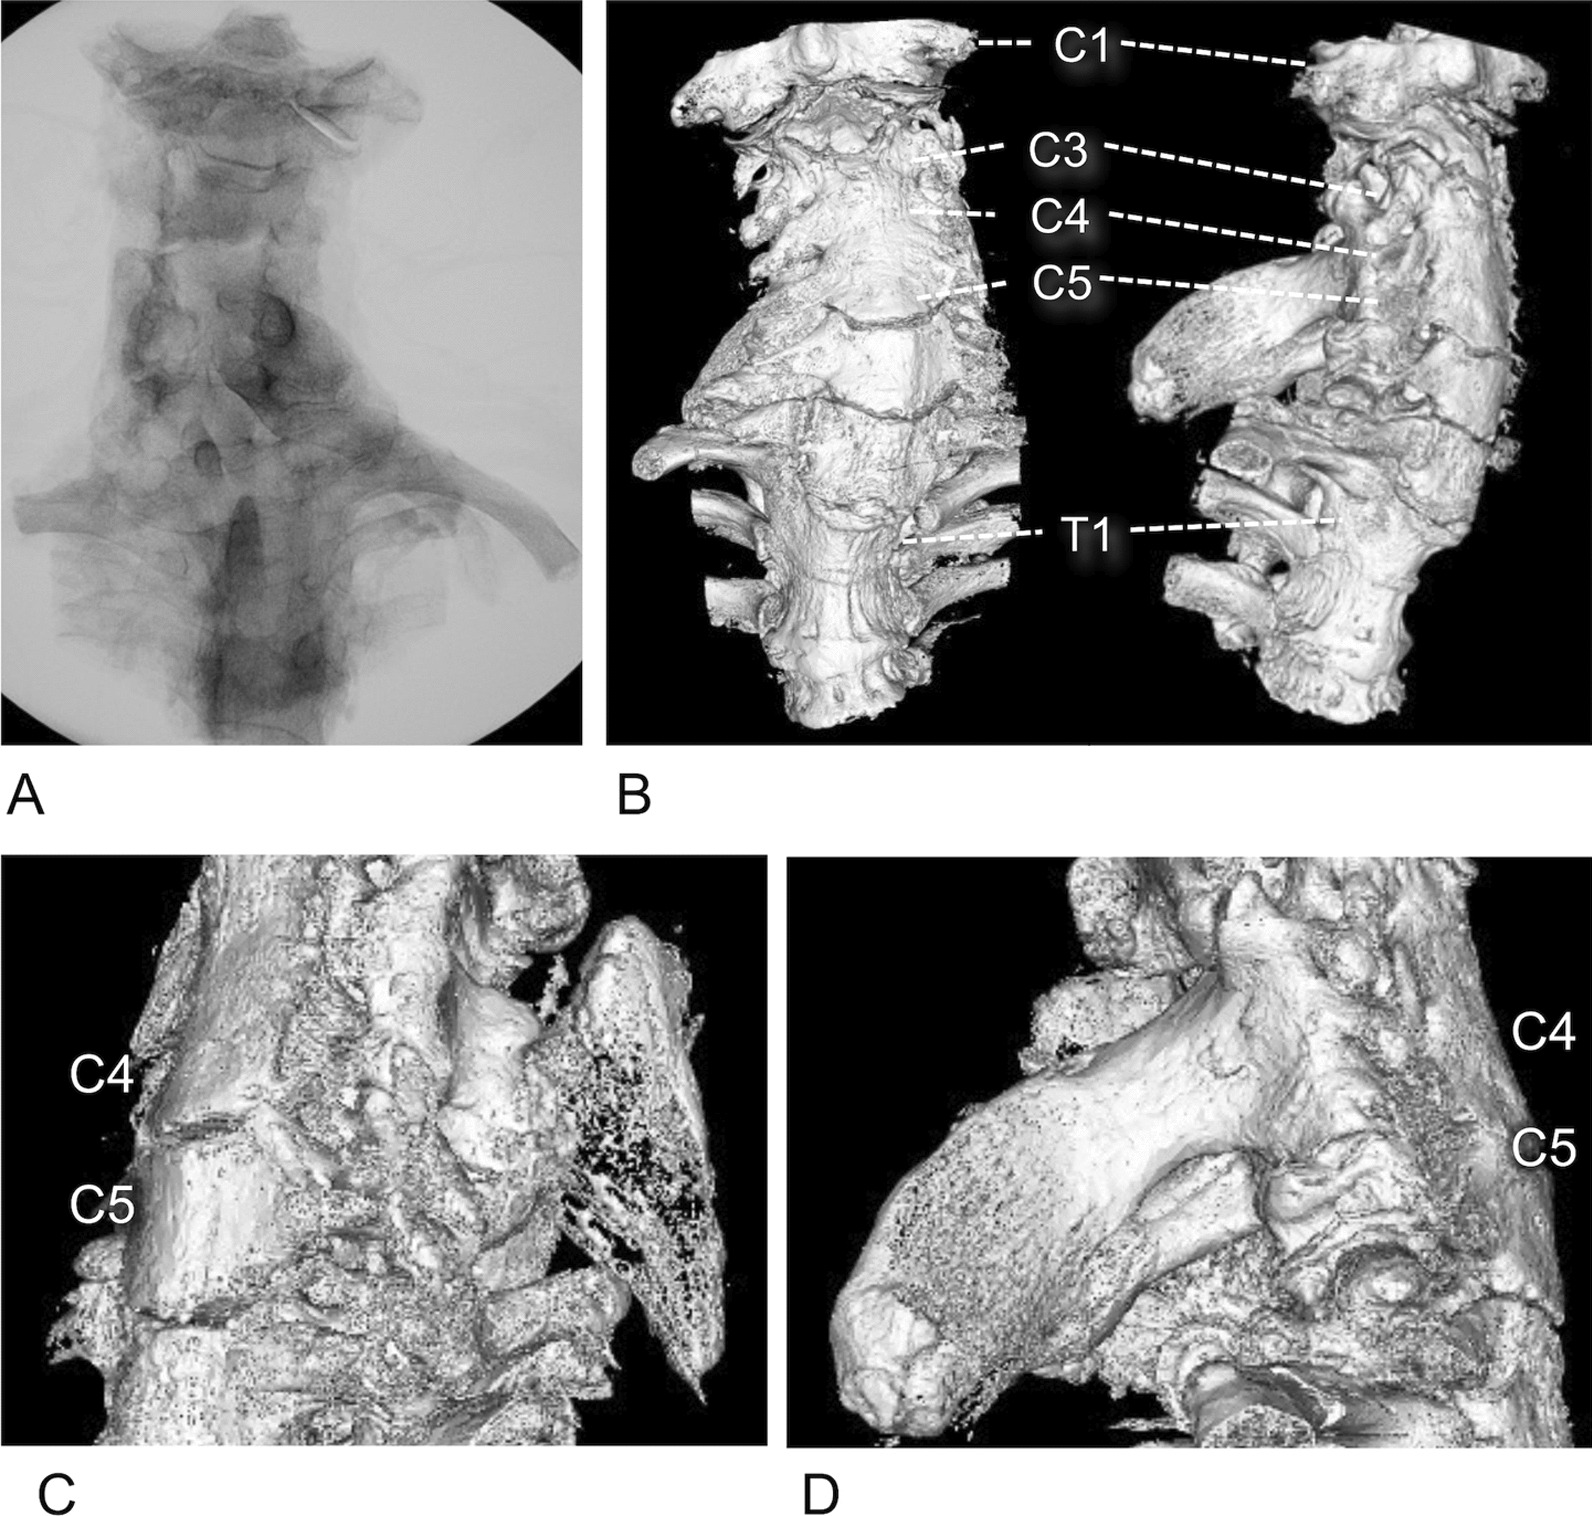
X-ray and computed tomography (CT) images of cervical and thoracic regions. A Posteroanterior X-ray image of the vertebrae (C1–T2). B Anterior and lateral views of the three-dimensional (3D) reconstructed CT scan of the vertebrae (C1–T2). Many vertebrae are deformed and fused. C and D 3D reconstructed CT images of the omovertebral bone on the left (C) and right sides (D)
